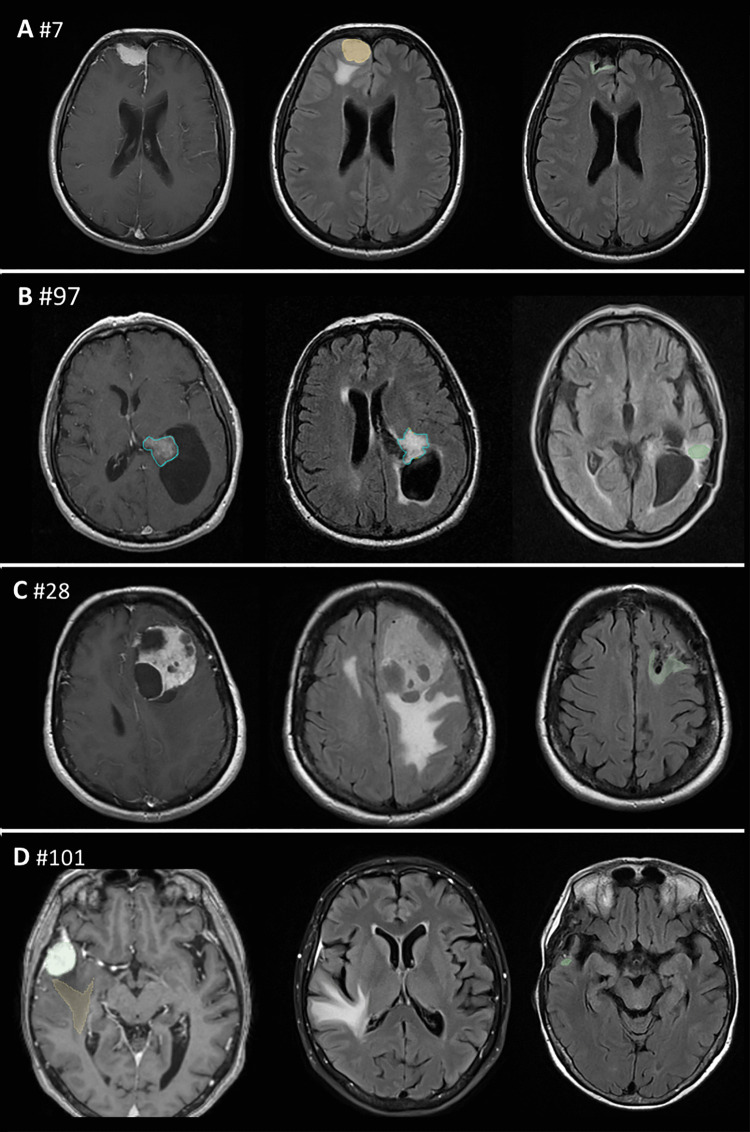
Axial MRI images of four meningioma cases: preoperative T1 contrast-enhanced (left), preoperative FLAIR (center), and postoperative FLAIR (right). Case 7(A): Grade II right frontal meningioma with near-complete PTBE resolution after gross total resection. Case 97(B): Grade I intraventricular meningioma in an atypical location, showing partial resolution of the periventricular edema and persistence of the edema on the temporal white matter. Case 28(C): Left frontal cystic Grade I meningioma with atypical enhancement and > 80% PTBE resolution post-surgery. Case 101(D): Tumor with homogeneous enhancement and a typical digitiform HI pattern, with more than 80% resolution postoperatively
